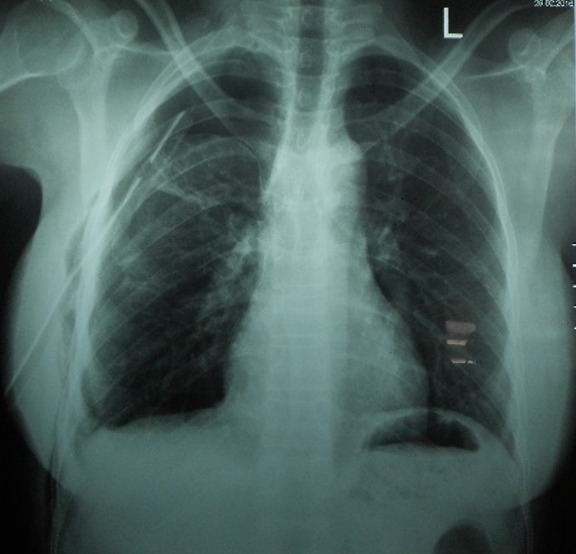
A repeat chest x-ray after 48 hours showing adequate re-expansion
